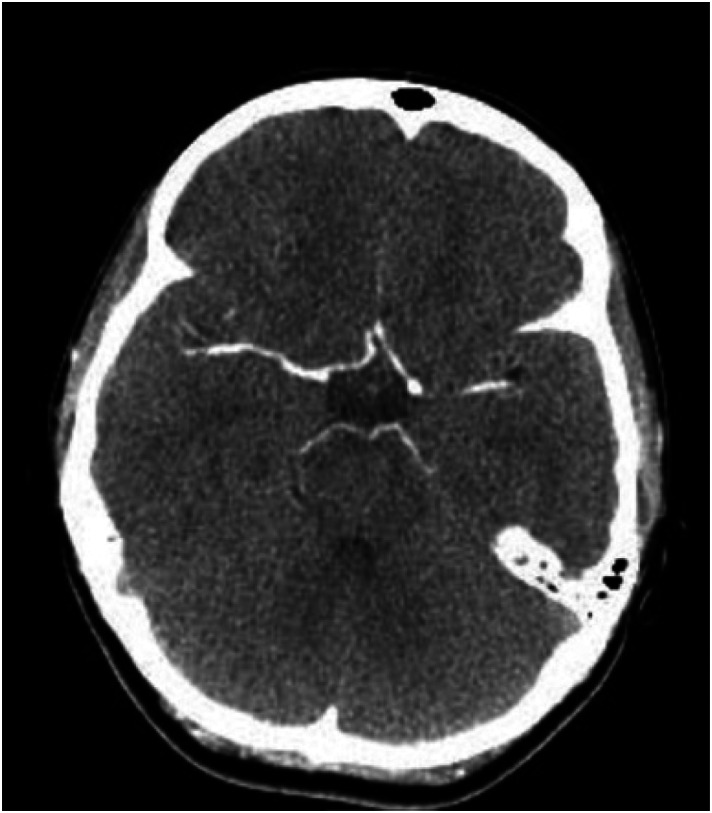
Normal computed tomography angiography at the level of the circle of Willis.
